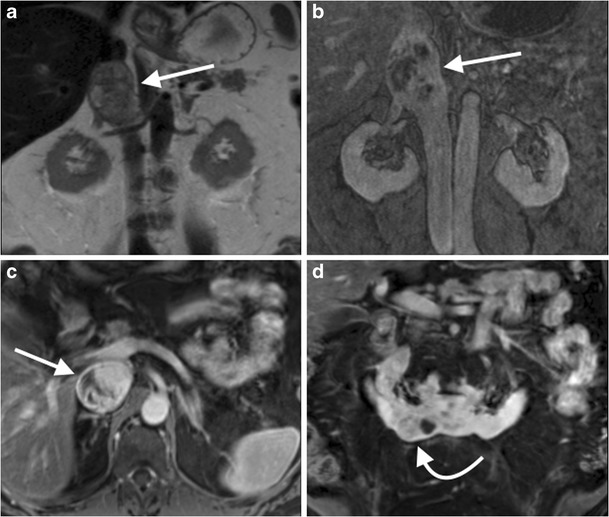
A 45-year-old man with bilateral leg swelling underwent an MR venogram of the abdomen and pelvis. Coronal image from a T2-weighted sequence (a) demonstrated an expanded suprarenal IVC with heterogeneous T2 high signal material, likely thrombus (*arrow*). Coronal (b) and axial (c) images from a venous phase T1-weighted fat-saturated post-gadolinium sequence demonstrates heterogeneous enhancement of the intraluminal IVC material, concerning for tumour thrombus. A coronal image from a T1-weighted fat-saturated post-gadolinium sequence of the abdomen (d) demonstrates a horseshoe kidney with an exophytic mass arising from the right lower moiety (*curved arrow*), which was subsequently confirmed as a papillary renal cell carcinoma on biopsy
